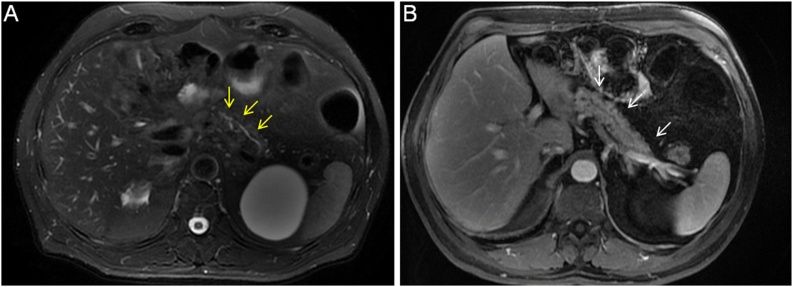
A: Axial fat-suppressed T2-weighted MRI sequence four years after surgery: main pancreatic duct with a normal caliber and without progression of the disease or new pancreatic cystic lesions (yellow arrows). B: Axial fat-suppressed T1-weighted MRI sequence: pancreatic remnant (white arrows).gr4
